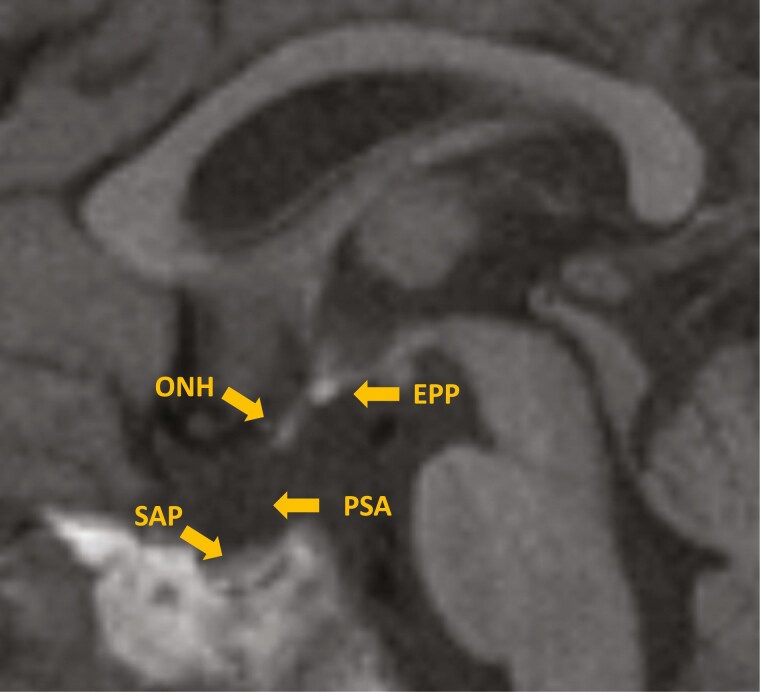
Brain magnetic resonance imaging—case 3. The image shows very small anterior pituitary, ectopic posterior pituitary, absent pituitary stalk, small optic nerves and chiasm. EPP, ectopic posterior pituitary; ONH, optic nerve hypoplasia; PSA, pituitary stalk absence; SAP, small anterior pituitary.
